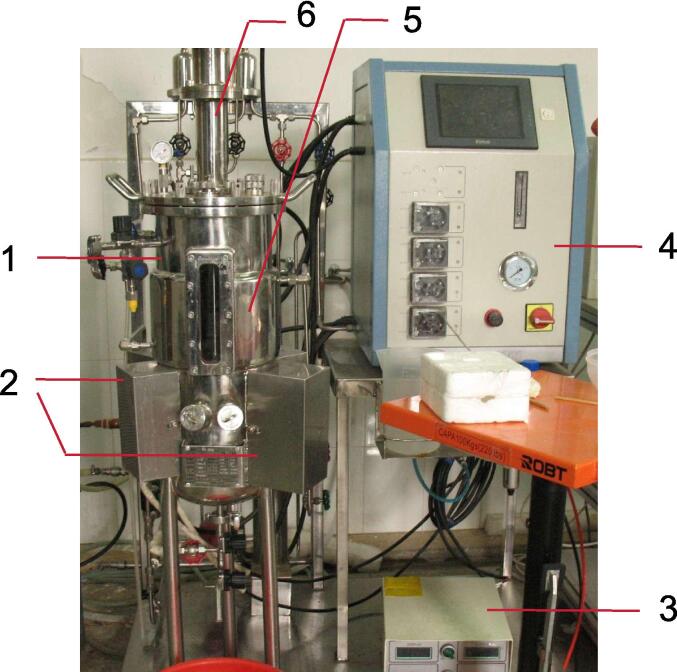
The 20 L Multi-Sweeping-Frequency Mode Ultrasonics Reactor 1- Reactor tank; 2- Sonicators chambers; 3- Ultrasonic generator; 4- Reactor Control Center; 5- Jacketed water bath temperature control system; 6- Mixing device.
